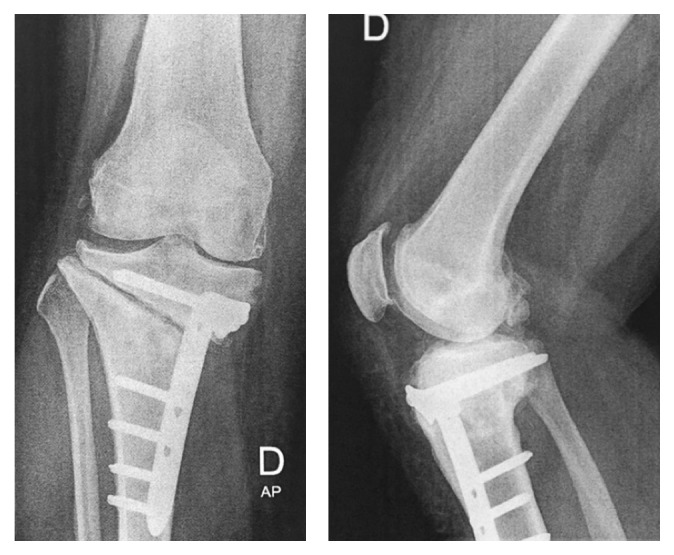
June 2012, 2 years after revision, postoperative X-rays showing medial gonarthritis.
